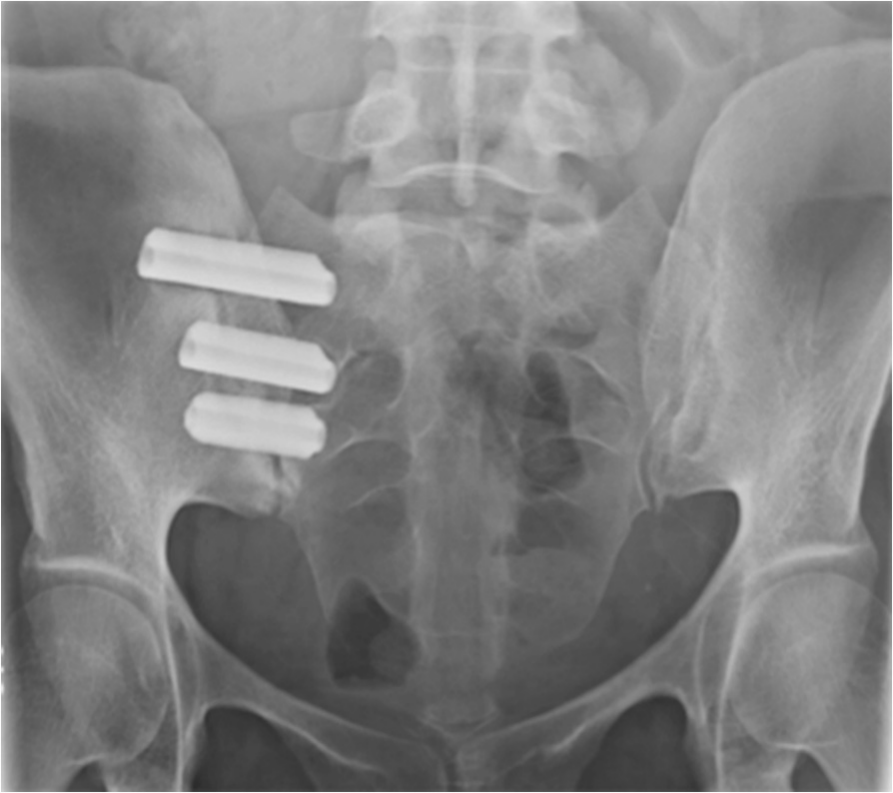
Postoperative radiograph demonstrating placement of three implants across the SI joint.
